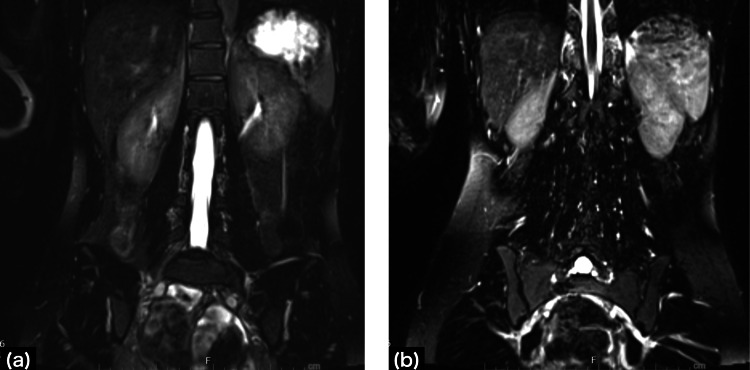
Magnetic resonance imaging T2-weighted images (a) Coronal view of the bilateral L5 root nerves; no calcareous deposits compressing nerve roots, including L5/S1, (b) coronal view of the gluteus maximus muscle level; there is no entrapment of the sciatic nerve in the deep gluteal muscles or anatomic variation of the sciatic nerve.
